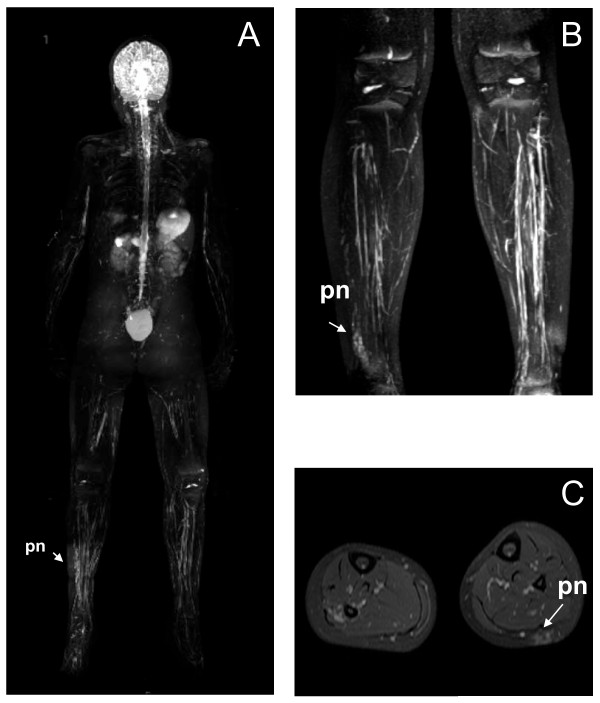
Whole body MRI of our first patient indicated a superficial plexiform neurofibroma (pn) of the left calf (A, B) and an internal plexiform neurofibroma above the right ankle (C).
